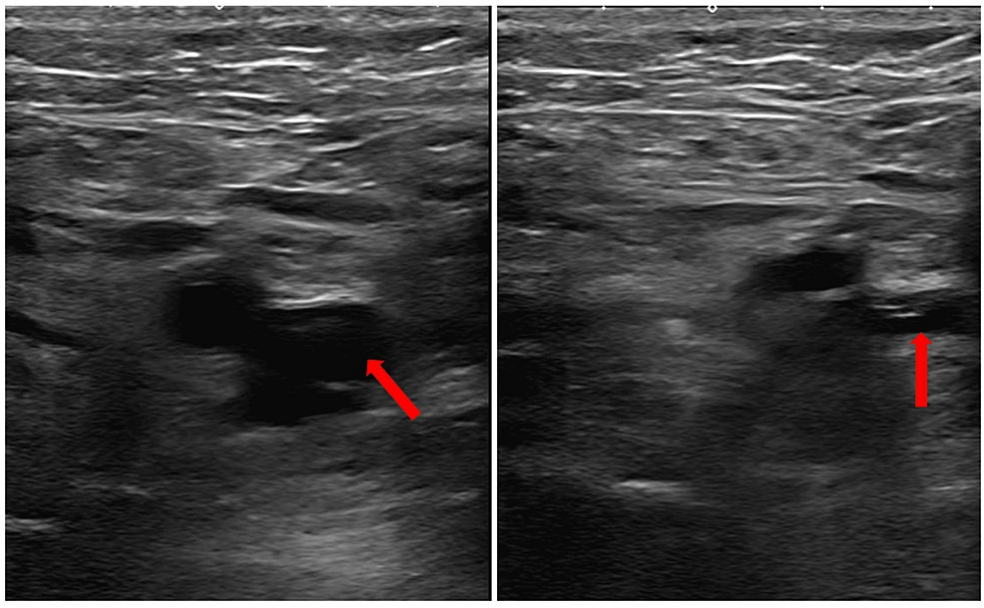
Duplex ultrasonography of the left lower extremity Venous system denoted by a red arrow with subsequent evidence of collapsibility
